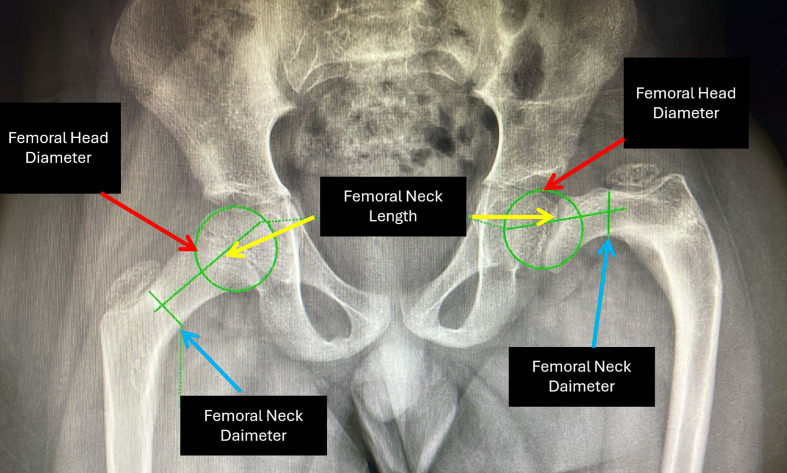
Femoral neck measurements on an anteroposterior (AP) pelvic radiograph.
